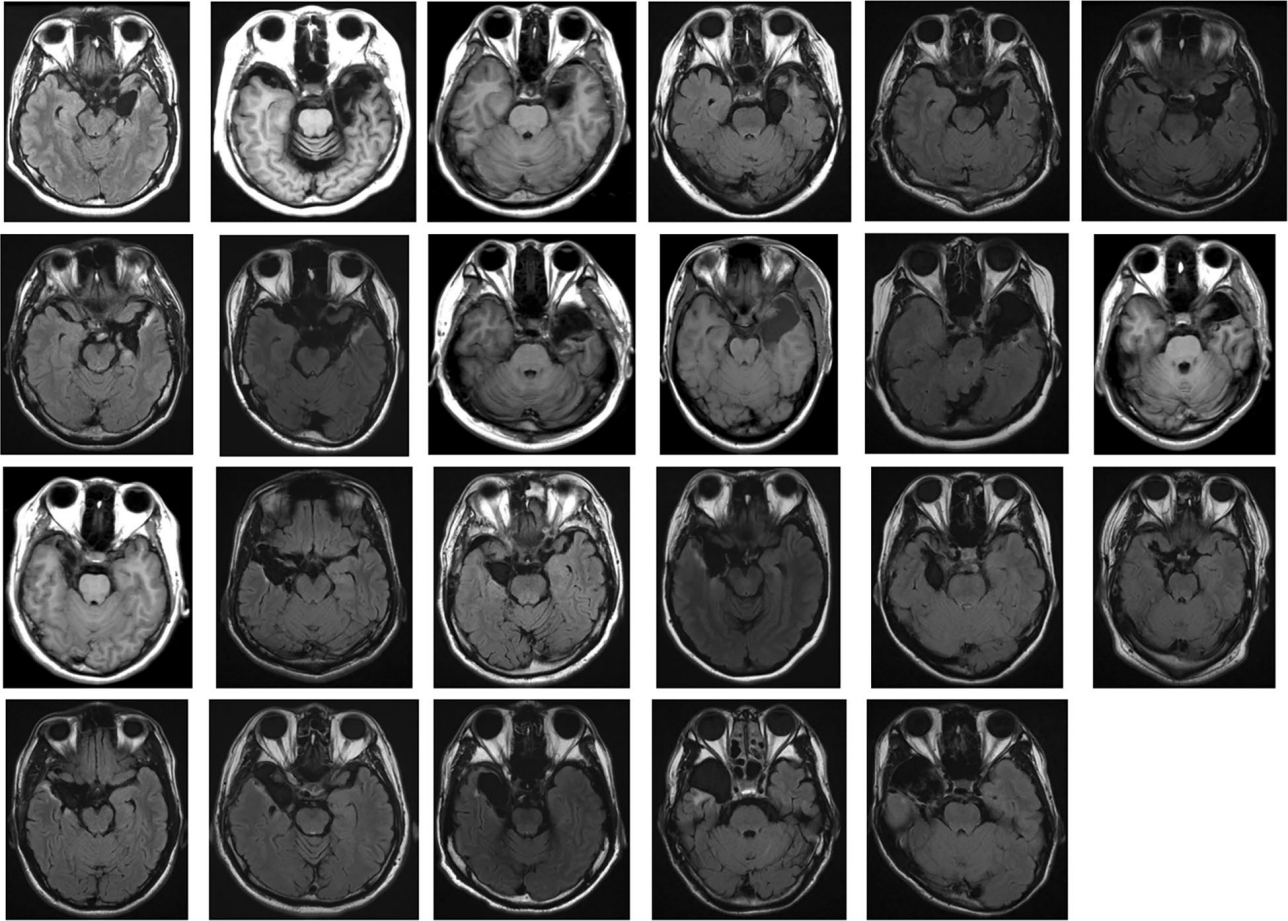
Anatomical magnetic resonance images of the temporal-lobe-resected patients. Left is shown on the right in the images.
